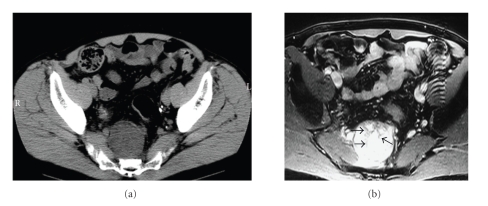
(a) CT showing a 6 cm diameter low-density tumor within the pelvis. (b) Axial T2-weighted MR image showing a very-high-intensity mass with a low-intensity septum (arrows).
